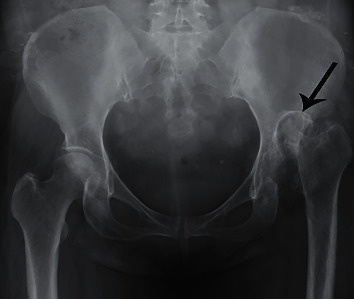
Anteroposterior X-ray images of the pelvis showed that the left femoral head had been absorbed, and the rest of the femoral neck had been dislocated and formed a pseudarthrosis (arrow).
